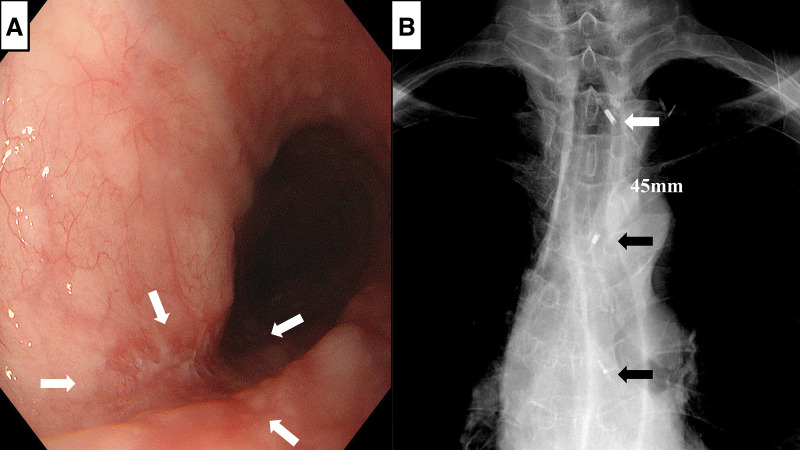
Upper gastrointestinal endoscopy and chest X-ray following endoscopic clipping. (A) Upper gastrointestinal endoscopy showing squamous cell carcinoma in the middle thoracic esophagus (arrowheads). (B) Marking clips were placed at the site of the jejunal–esophageal anastomosis (white arrowhead) and on both the proximal and distal sides of the esophageal carcinoma (black arrowheads). The distance between the jejunal–esophageal anastomosis and the proximal side of the esophageal carcinoma was approximately 45 mm.
